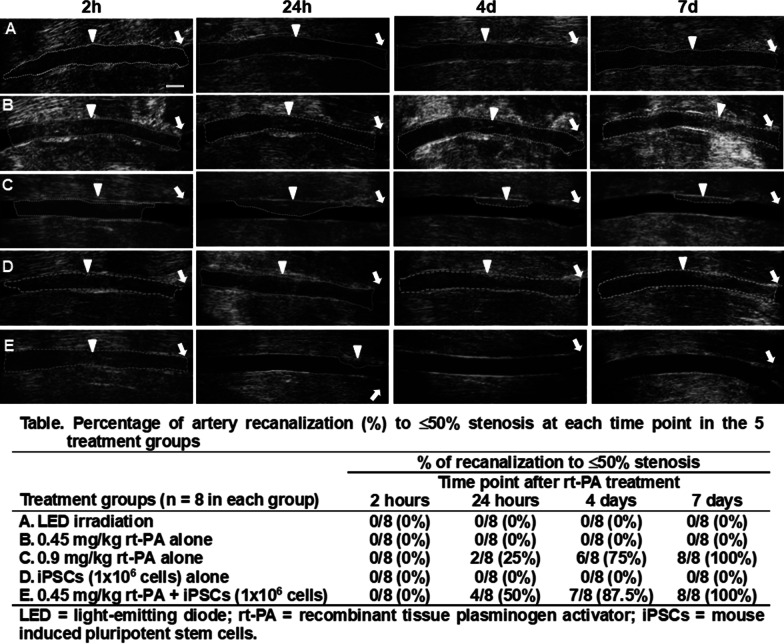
Ultrasound studies of common carotid artery (CCA) and the ratio of recanalization to ≤ 50% stenosis in the 5 treatment groups at 4 time points after 6 mW/cm^2^ LED irradiation for 4 h. CCD lumen is completely occluded at A, B and D groups from 2 h, 24 h, 4 days to 7 days. However, CCA lumen is occluded at 2 h but progressively recanalized from 24 h, 4 days to 7 days at C and E groups. A  LED irradiation alone (LED group), B LED irradiation plus intravenous bolus injection of 0.45 mg/kg rt-PA alone (0.45 mg/kg rt-PA group), C  LED irradiation plus intravenous bolus injection of 0.9 mg/kg rt-PA alone (0.9 mg/kg rt-PA group), D LED irradiation plus intravenous bolus injection of 1 × 10^6^ iPSCs alone (iPSCs group), E LED irradiation plus intravenous bolus injection of 0.45 mg/kg rt-PA and 1 h later, bolus injection of 1 × 10^6^ iPSCs (0.45 mg/kg rt-PA plus iPSCs group). LED = light-emitting diode; n = 8 in each group. Arrow indicates carotid bifurcation. Arrowhead and dot-line indicate the stenosis segment. h = hour; d = day. Scale bar in A (2 h) = 1 mm
